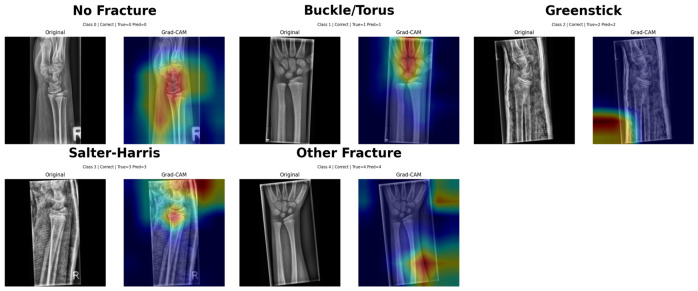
Gradient-weighted class activation mapping (Grad-CAM) saliency: DenseNet-169. Representative activation maps overlaid on correctly classified wrist radiographs for each fracture class. Model attention localizes to the distal radial metaphysis, physeal plate, and cortical margins, consistent with expected radiographic fracture localization. Warmer colors (red/yellow) indicate regions of higher model attention; cooler colors (blue) indicate lower attention.
